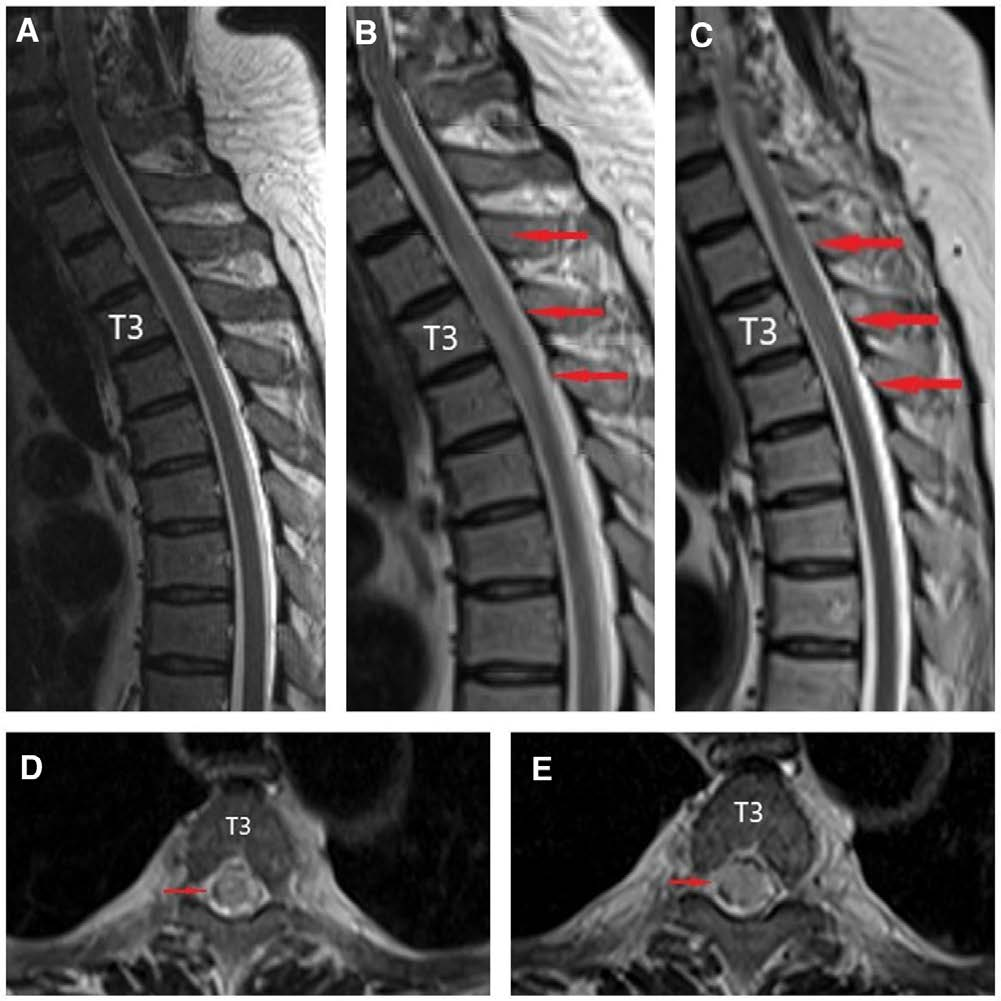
(A) MRI of the thoracic vertebra showed no cord injuries. Sagittal (B) and Axial (D) MRI of the thoracic vertebra demonstrated slight thickening of the 3rd to 5th thoracic spinal cord with increased T2 signal (red arrow). Sagittal (C) and Axial (E) MRI of the thoracic vertebra confirmed no obvious change compared with imaging in (B and D). MRI = magnetic resonance imaging.
